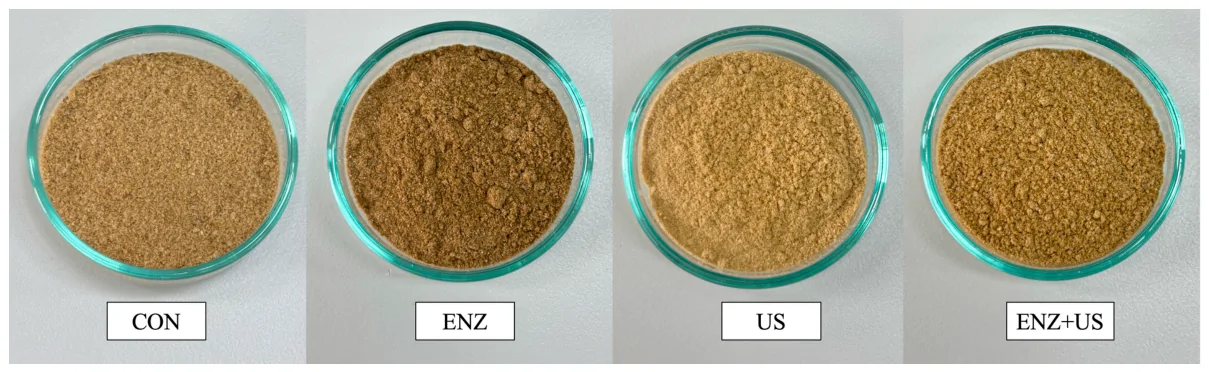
Mustard proteins extracted by different methods. CON, conventional alkaline extraction; ENZ, enzyme-pretreated alkaline extraction; US, ultrasound-assisted alkaline extraction; ENZ+US, enzyme-pretreated and ultrasound-assisted alkaline extraction.
